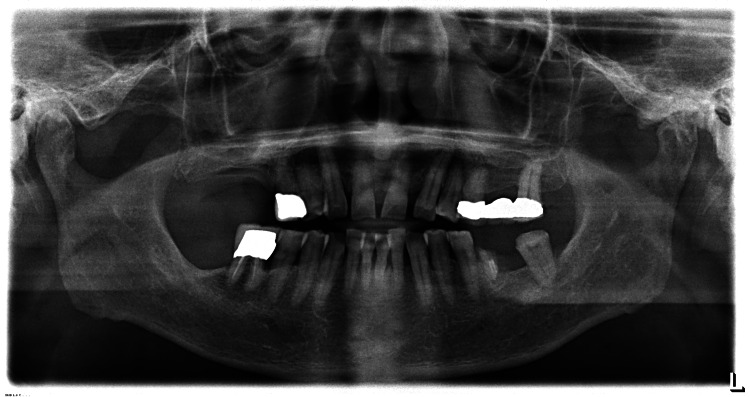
Panoramic radiograph showing generalized moderate to severe periodontitis A radiograph taken in November 2022 demonstrating generalized horizontal alveolar bone loss. The alveolar bone crest is positioned approximately 3-4 mm apical to the cementoenamel junction in most areas, indicating moderate to severe bone loss. These findings are consistent with the clinical diagnosis of generalized moderate to severe periodontitis
